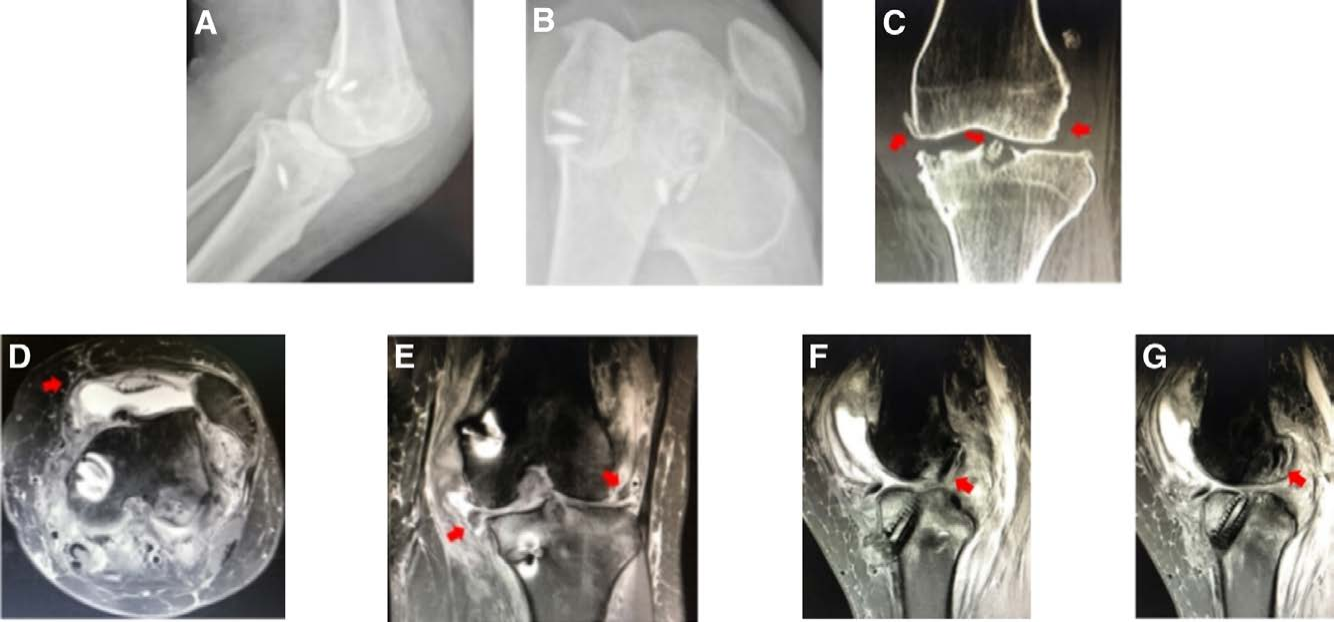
(A and B) DR findings: the left knee joint exhibited subluxation, a posterior tibia drop, and external patella dislocation. (C) CT imaging revealed external patella dislocation of the left knee, obvious degeneration and hyperplasia of the knee joint, the presence of a tibial tunnel extrusion screw protruding into the joint cavity, and subluxation of the knee joint. (D) MRI findings: medial patellofemoral retinacular tear of the left knee. (E) MRI findings revealed subluxation of the left knee joint, a medial collateral ligament tear, medial and lateral meniscus dislocation, and the presence of a tibial tunnel extrusion screw protruding into the joint cavity. (F and G) MRI results: the reconstructed ACL injury of the left knee was edematous; however, some tendons were still visible. ACL = anterior cruciate ligament.
